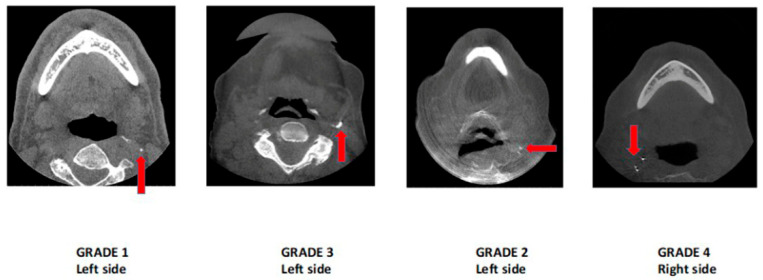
Axial-section CBCT images demonstrating unilateral ECACs according to the proposed grading scheme.
